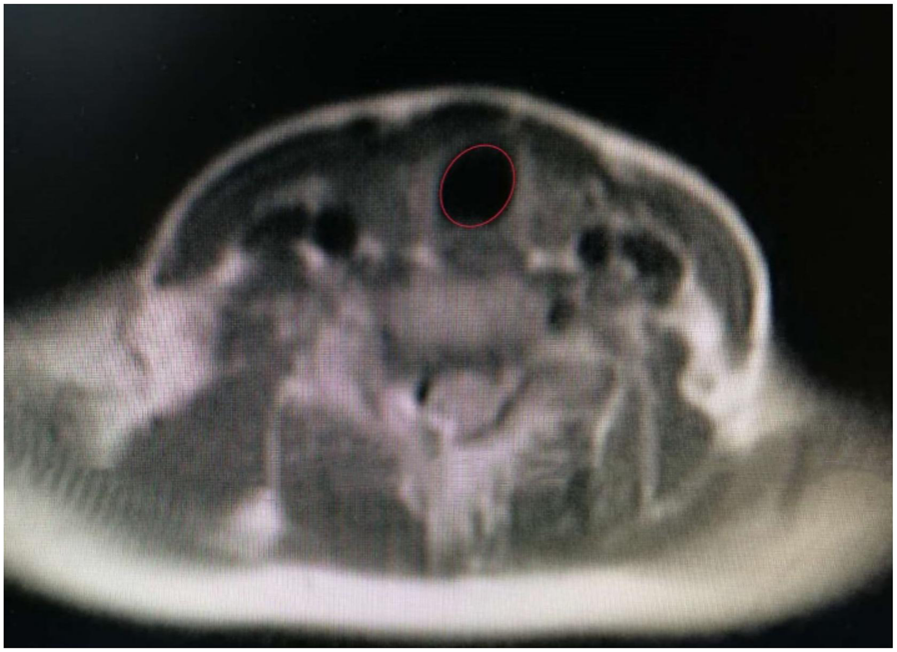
The anatomy of the neck of the pediatric patients with cervical lateral bending in MRI. The red circle represents the cricoid cartilage
